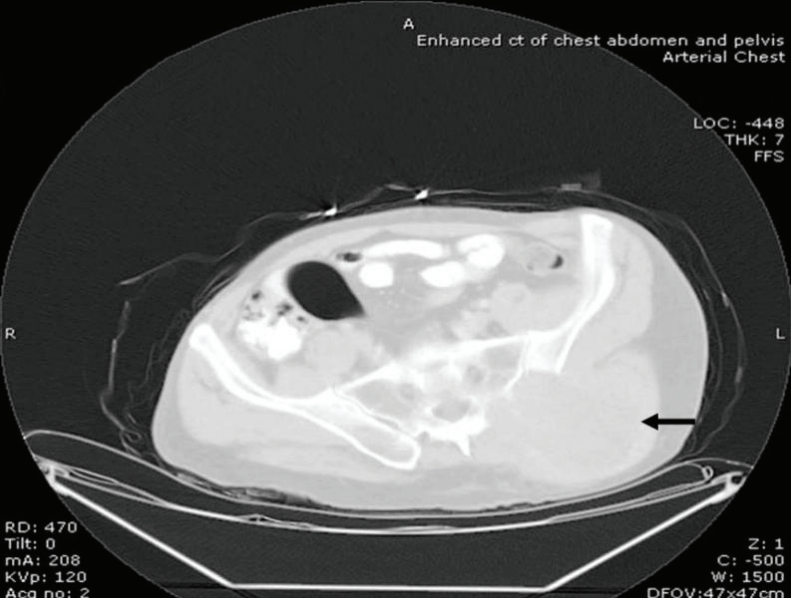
CT scan of large soft tissue mass in the posterior sacro-iliac joints (black arrow).
